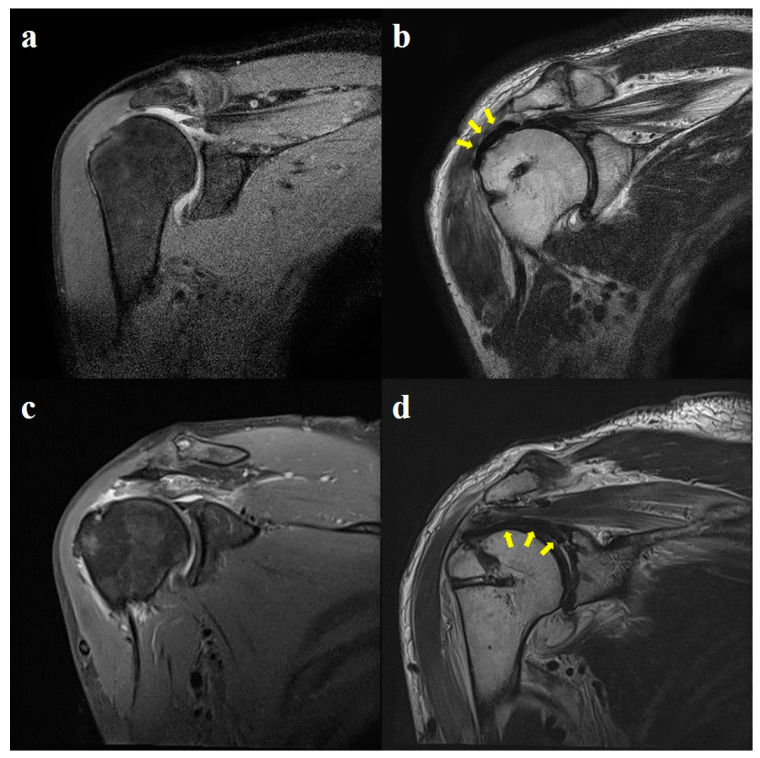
Preoperative and postoperative MRI images. (a,b) LMRCTs are shown in the preoperative MRI image. In the postoperative MRI image, LMRCTs have been repaired, and the patch (yellow arrows) fully covers the defect and the greater tuberosity using PGA reinforcement. (c,d) LMRCTs are shown in the preoperative MRI image. In the postoperative MRI image, SCR using a patch (yellow arrows) was performed, and this fully covered the greater tuberosity. LMRCTs were well repaired over the patch. LMRCTs, large-to-massive rotator cuff tears; MRI, magnetic resonance imaging; SCR, superior capsule reconstruction; PGA, patch graft augmentation.
